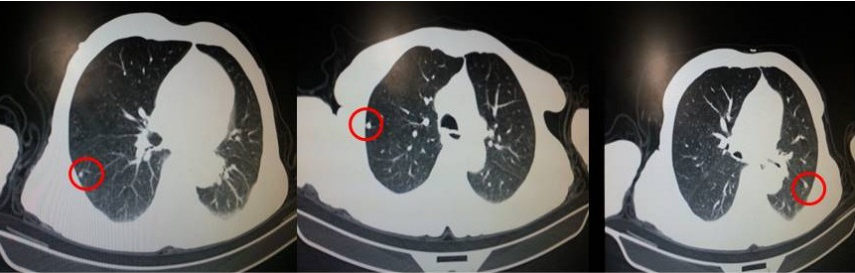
Thoracic computed tomography showing bilateral suspicious lung nodules
